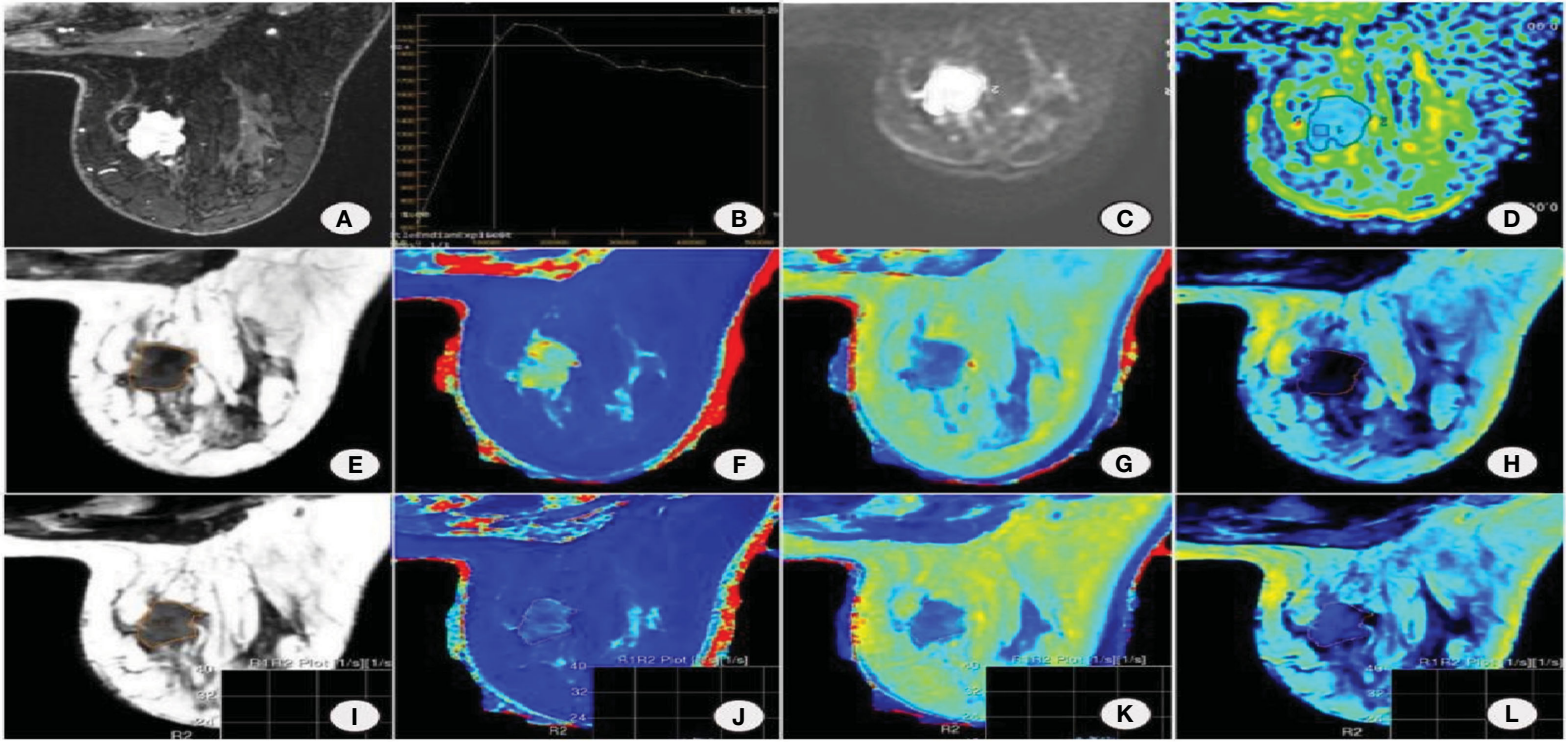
Female, 53 years old with non-special invasive carcinoma of the right breast. (A) DISCO enhancement image showing irregular mass with irregular margin and uneven enhancement in the upper inner quadrant of the right breast. (B) TIC is a washout. (C) DWI shows mass diffusion limitation. (D) ADC pseudocolor map. Image reconstruction with MAGiC. (E) SyMRI T2WI. (F) T1 map. (G) T2 map. (H) PD map. (I) SyMRI T2WI enhancement. (J) T1 enhancement map. (K) T2 enhancement map. (L) PD enhancement map.
